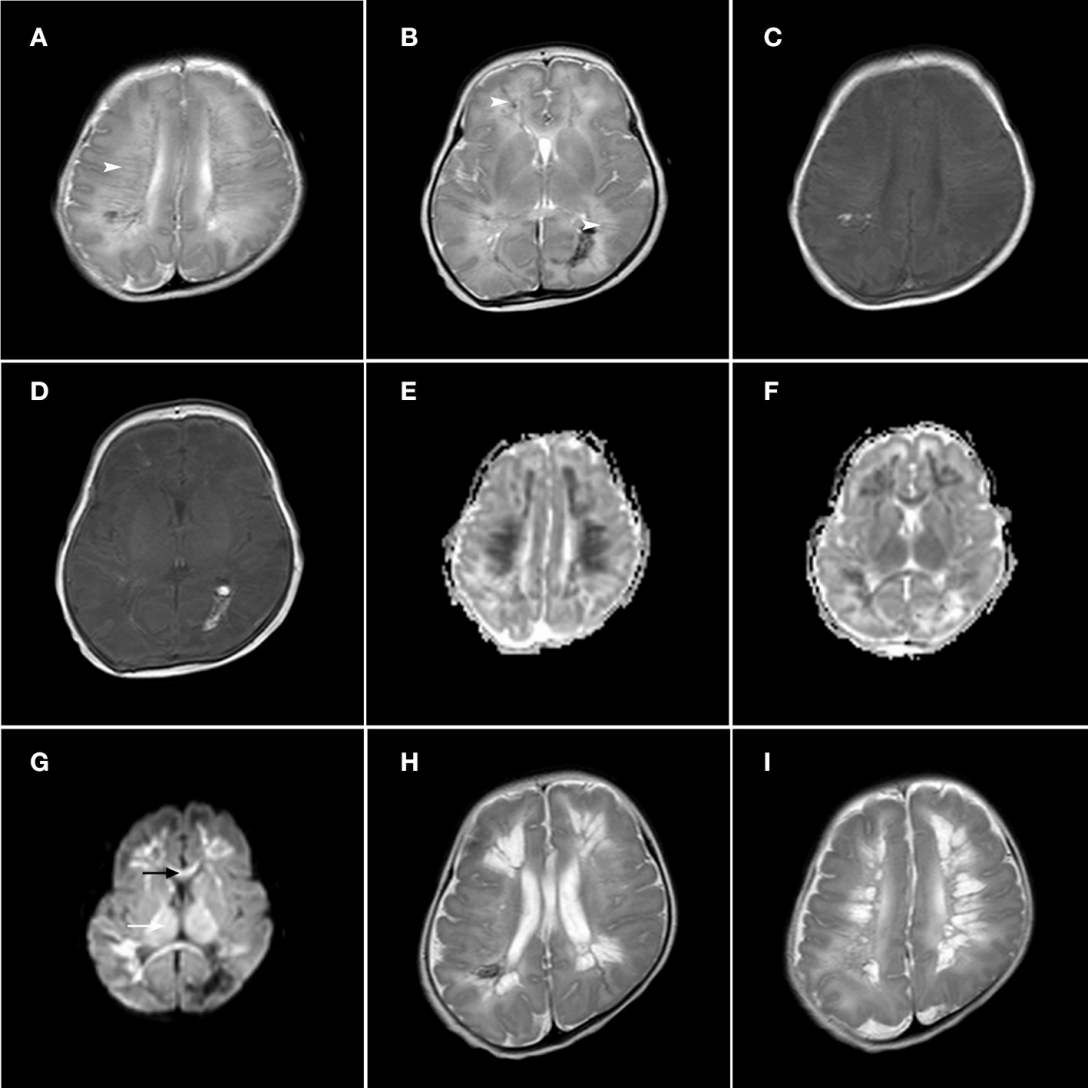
Axial T1-weighted image, axial T2-weighted image, axial ADC maps and DWI sections in a late premature neonate (gestational age, 35+5 weeks; (A–G): 8 days after birth, (H,I): 21 days after birth). The T2-weighted images show linear and radial lesions in the frontal parietal area (A) and prefrontal area and peritrigonal area (B), with low signal changes (arrow head). The T1-weighted signal did not change significantly (C,D). Axial ADC maps showed a significant decrease in ADC value in the white matter area around the linear injury (E,F). In DWI (G), the signals of the corpus callosum (black arrow) and thalamus (white arrow) were increased. On the 21st day after birth, the second T2-weighted MRI scan showed the formation of cystic changes in the prefrontal region, trigonal region (H) and frontal parietal region (I).
